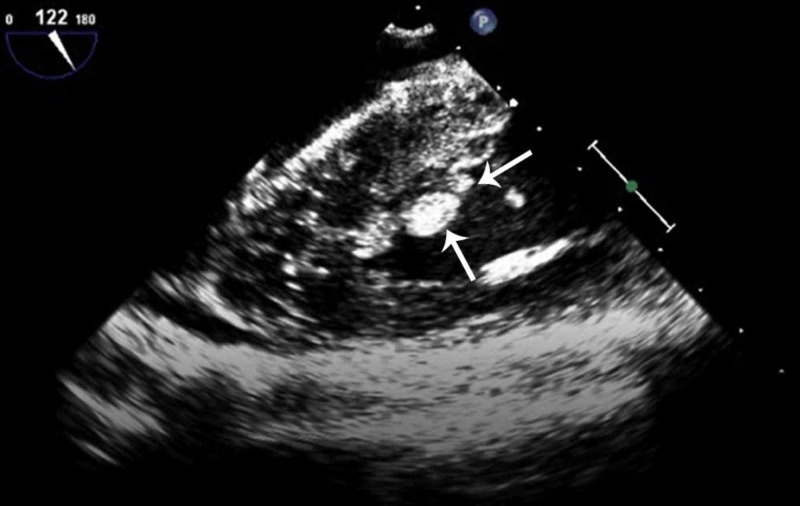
Transesophageal echocardiogram image showing the right ventricle and the tricuspid valve. Two of the four echogenic densities/ masses attached to the chordae are also seen (white arrows).
